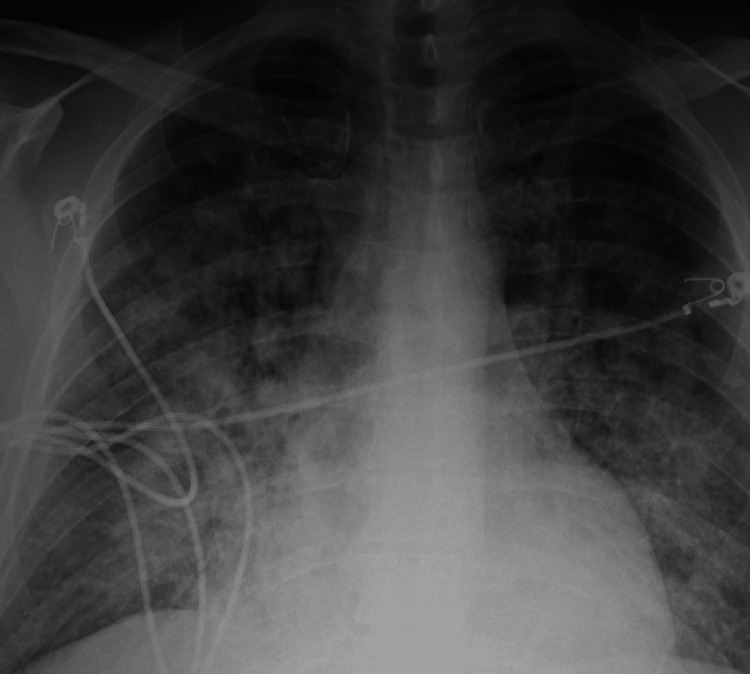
Portable chest x-ray revealing acute pulmonary edema
